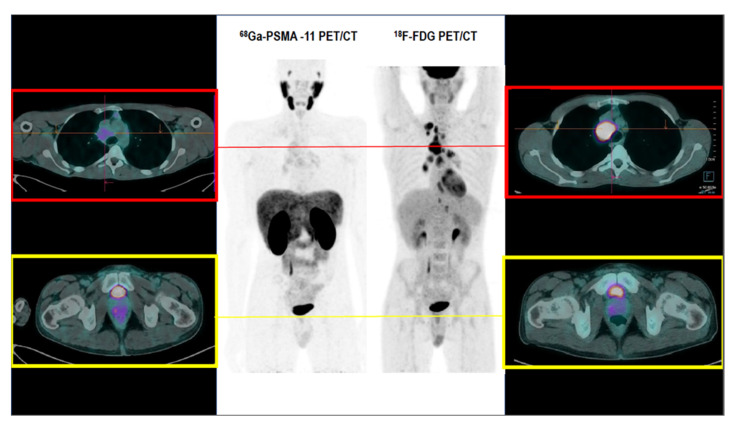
The central panel displays the Maximum Intensity Projection (MIP) of 68Ga-PSMA-11 and 18F-FDG PET/CT scans. The left axial hybrid PET/CT images show the focal 68Ga-PSMA uptake at the peripheral zone of the right prostatic lobe (yellow) and the mild tracer uptake by enlarged mediastinal lymph nodes (red). The right axial hybrid PET/CT images show the high 18F-FDG uptake by the enlarged mediastinal lymphatic stations (red) and the low 18F-FDG uptake by the peripheral zone of the right prostatic lobe (yellow).
